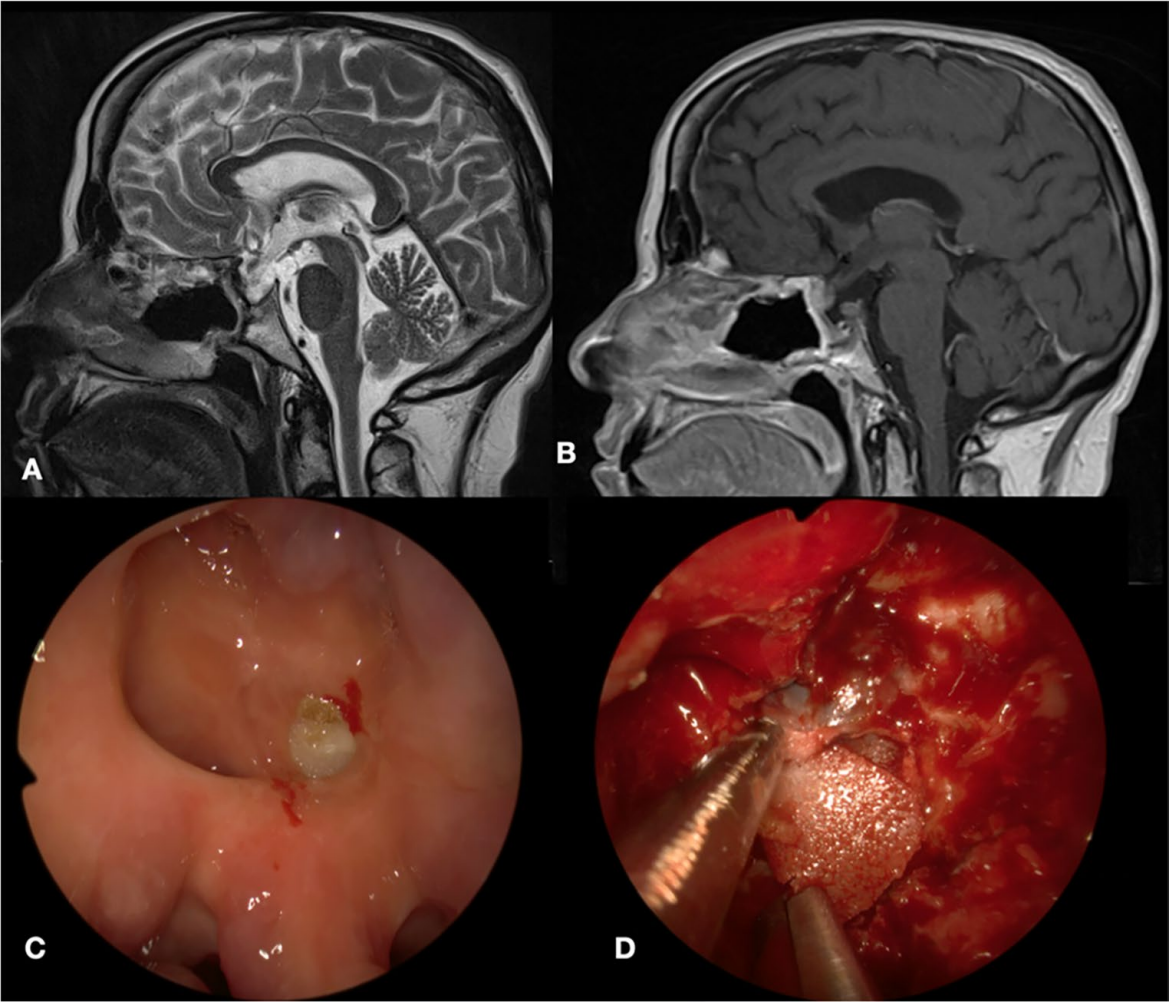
Intraoperative findings. A, B: Pre-operative T2 and T1 weighted brain MRI showing the ipointense Medpor. C: Intraoperative images with evidence of Medpor exposure through a mucosal defect. D: Intraoperative image of Medpor’s removal
